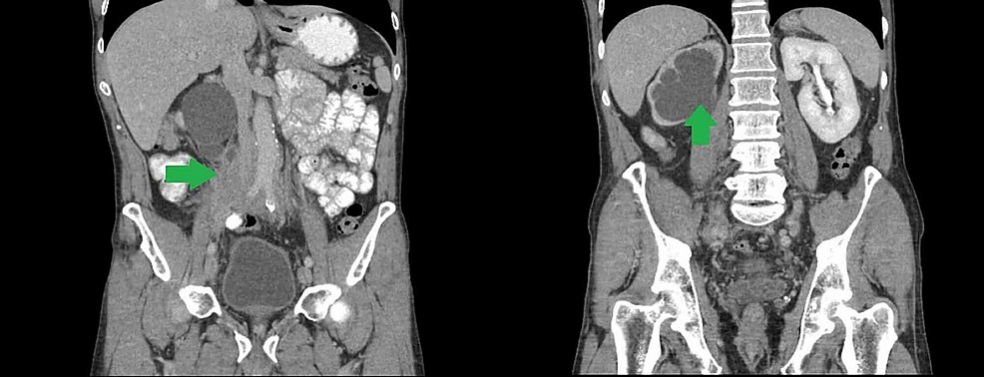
Initial abdominal CT scan. Left: predominantly right-sided retroperitoneal mass encircling the aorta and inferior vena cava. Right: Massive distention of the right pyelocaliceal system and marked loss of renal cortex. CT, computed tomography
